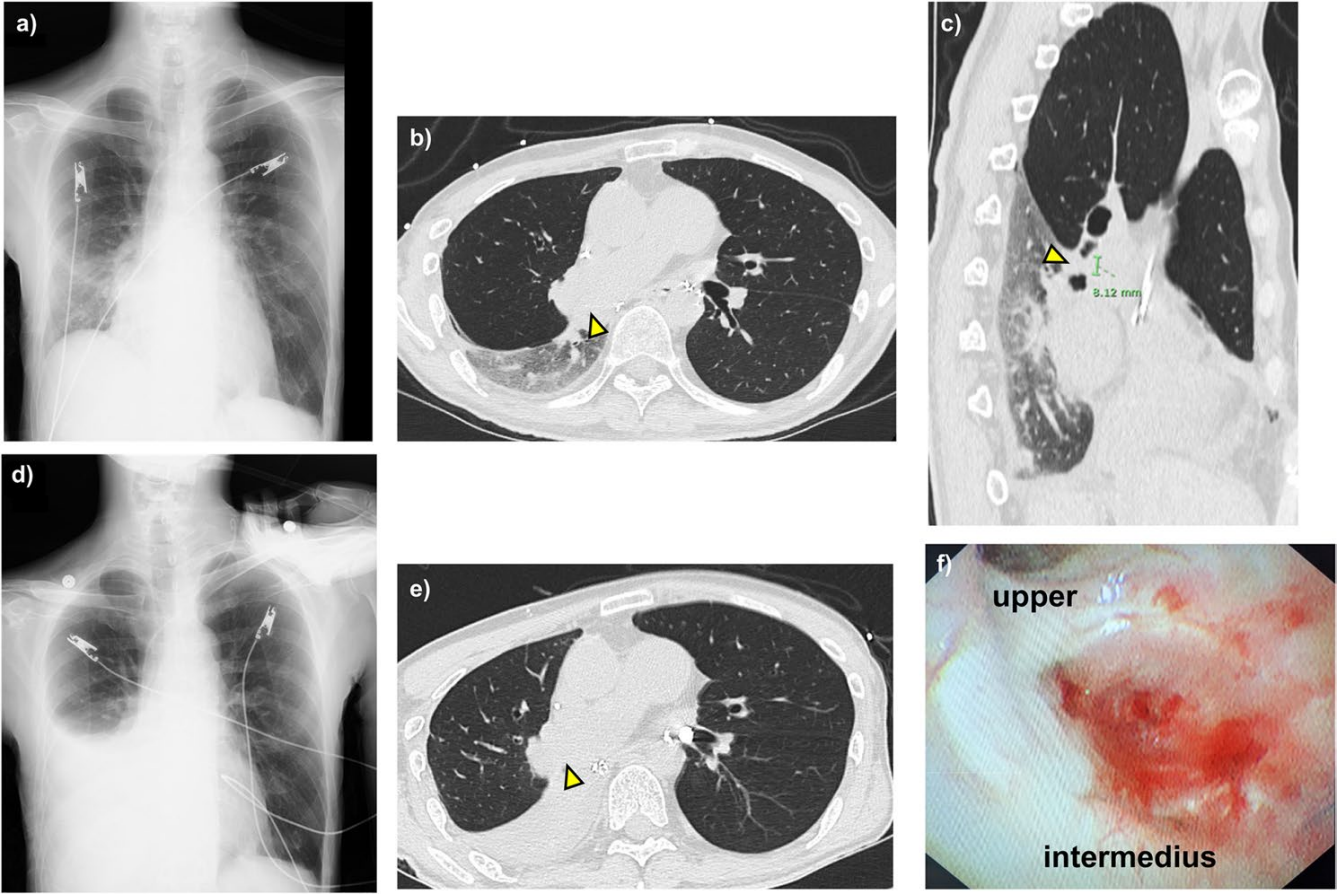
Radiological and bronchoscopic findings at the onset of respiratory deterioration 5 months after lung transplantation. a Chest radiography at initial presentation showing mildly decreased lucency and slight elevation of the right hemidiaphragm. b Chest radiography obtained after further deterioration demonstrating marked opacity of the right lower lung field. c, d Computed tomography images showing nearly complete obstruction of the bronchus intermedius (yellow arrows). The obstructed segment measured approximately 0.8 cm in length, with residual distal aeration and reduced transparency of the right middle and lower lobes. e Follow-up computed tomography obtained on the following day showing complete obstruction of the bronchus intermedius (yellow arrow) and total atelectasis of the middle and lower lobes. f Bronchoscopic findings show complete obstruction of the bronchus intermedius
